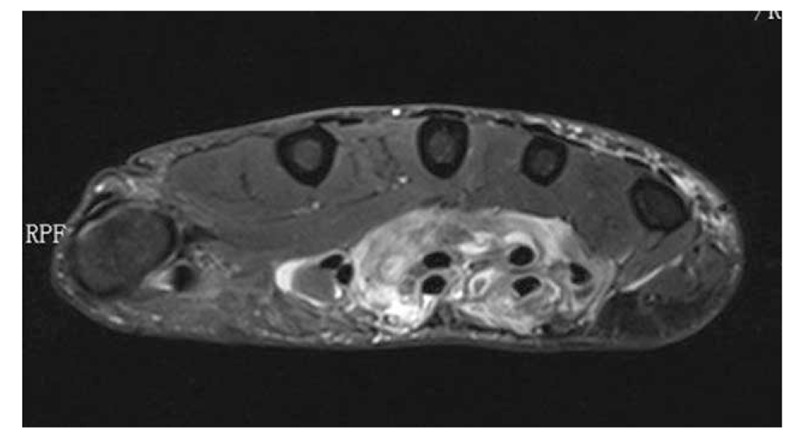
High signal in area of wrist in T2-weighted sequences of MRI. MRI = magnetic resonance imaging.
